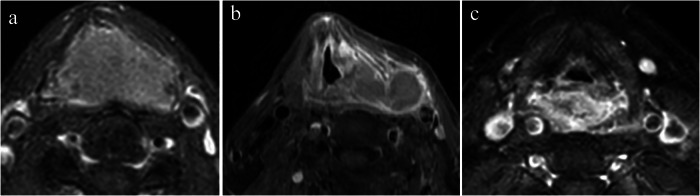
Examples for T1-weighted contrast enhancement patterns. Homogeneous enhancement is defined as a uniform and even distribution of contrast material on contrast-enhanced T1-weighted MR images, with minimal heterogeneity or necrosis (a). Homogeneous enhancement with an outer ring sign (b) is characterized by a uniformly enhanced central region (homogeneous enhancement) surrounded by a well-defined, hyperintense peripheral rim (outer ring sign). Heterogeneous enhancement (c) is characterized by a non-uniform distribution of signal intensity on contrast-enhanced T1-weighted MR images
